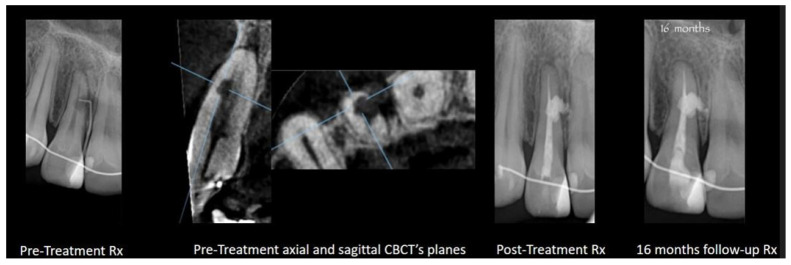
Maxillary lateral incisor with external cervical resorption (with a wide subcrestal entry point) obturated with GP and TSS. X-ray images of pre- and post-treatments and from a 16-month follow up.
